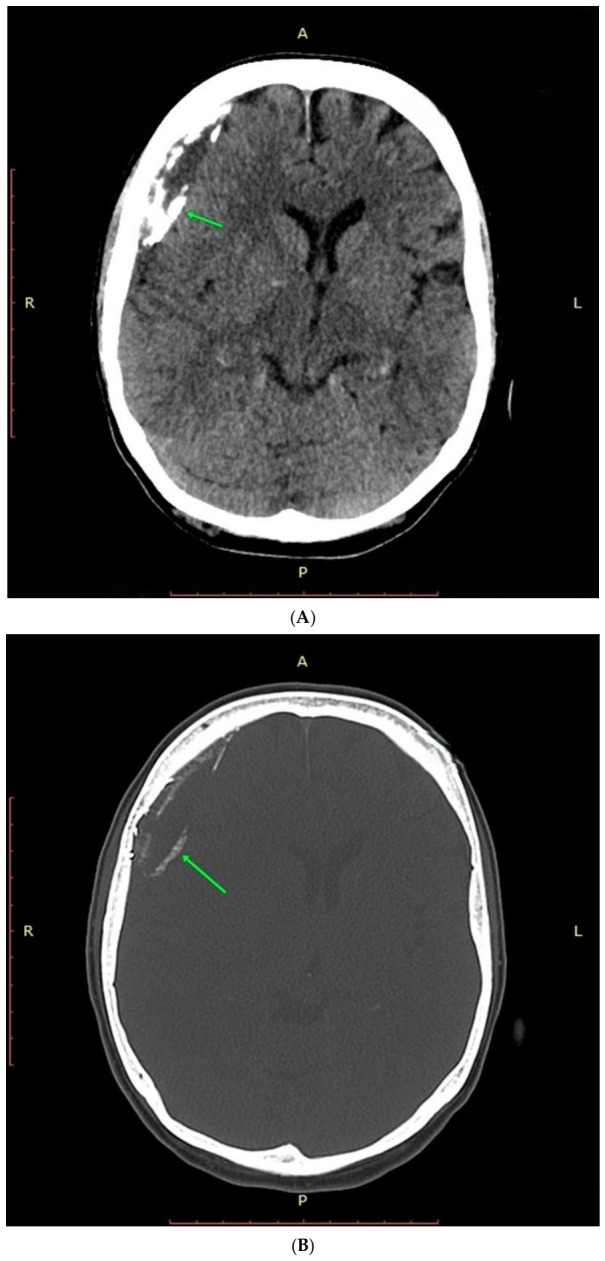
Axial section of the brain on non-contrast CT in both the standard window (A) and bone window (B) (within the hematoma capsule, a hyperdense focus is visible, indicated by an arrow, corresponding to deposited embolic material—20% histoacryl glue [n-butyl cyanoacrylate, n-BCA]). Own material. A—Anterior; L—Left; P—Posterior; R—Right.
